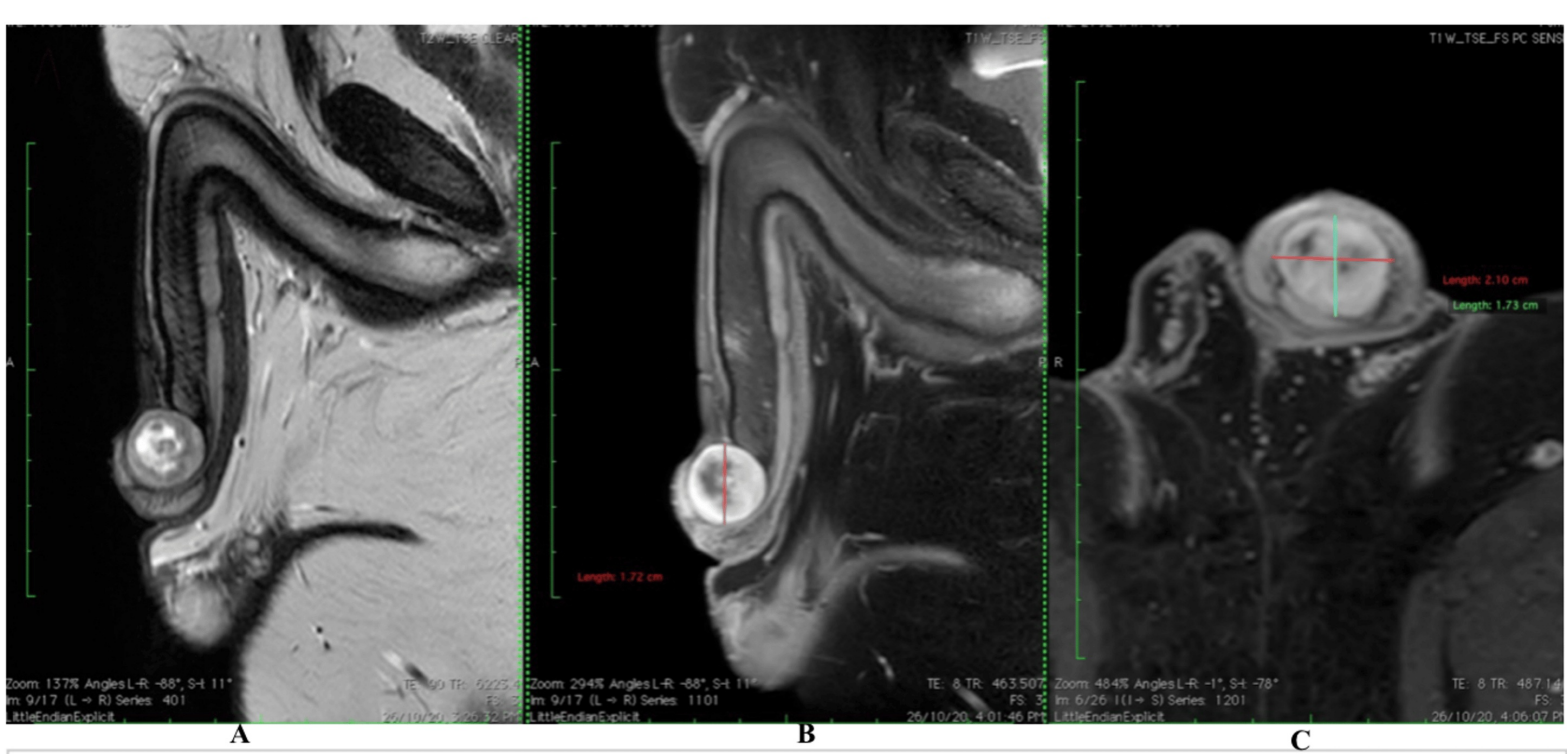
MRI of the penis showing a well-circumscribed glans mass (A) Sagittal T2-weighted image showing a well-circumscribed ovoid mass within the glans with heterogeneous signal intensity and internal hyperintense cystic/necrotic areas. (B) Sagittal post-contrast T1-weighted fat-suppressed image demonstrating avid enhancement of the lesion. (C) Axial post-contrast T1-weighted fat-suppressed image showing the enhancing mass abutting but not invading the corpora cavernosa, corpus spongiosum, and penile urethra.
